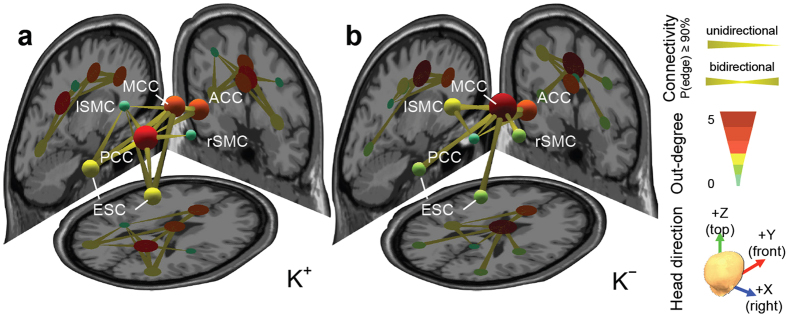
Brain network dynamics. Effective connectivity between EEG independent processes estimated under (a) K^+^ and (b) K^−^ conditions. Three-dimensional plot is formed with three anatomical MRI slices (left: sagittal view; right: coronal view; bottom: horizontal view) as background. Node represents anatomical location of each independent process, localized using a single, or dual-symmetric equivalent-current dipole model based on a four-shell spherical head model. Color of nodes represents degree of outflow. Edges represent causality directions. P(edge) ≥ 0.9 indicates that over 90% of participants have a significantly non-zero connectivity magnitude in that edge (see Supplementary Table 3). Out-degree indicates the number of outgoing edges.
